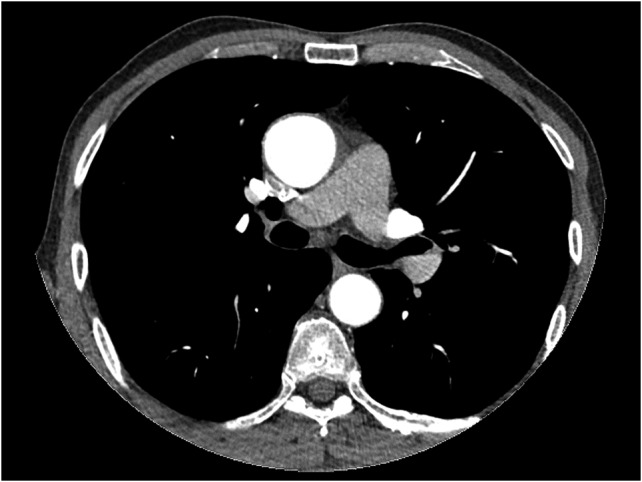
Thorax CT angiography showing the dilatation of ascending aorta of a patient before surgery on admission to our center.
